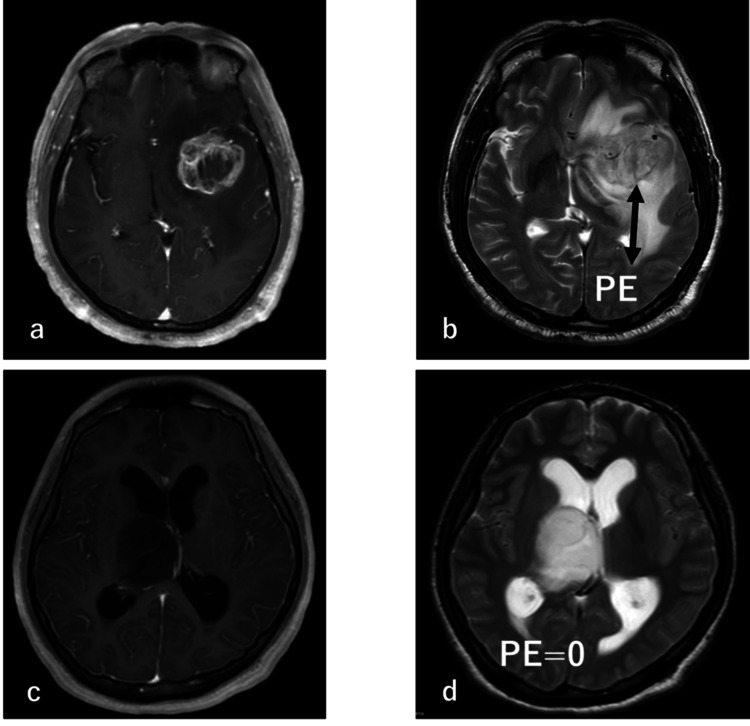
Definition of preoperative edema in cases with and without contrast enhancement. (a) Contrast-enhanced T1-weighted MRI in a case with contrast enhancement. (b) T2-weighted MRI in the case in (a). (c) Contrast-enhanced T1-weighted MRI in a case without contrast enhancement. (d) T2-weighted MRI in the case in (c). PE: preoperative edema; MRI: magnetic resonance imaging
